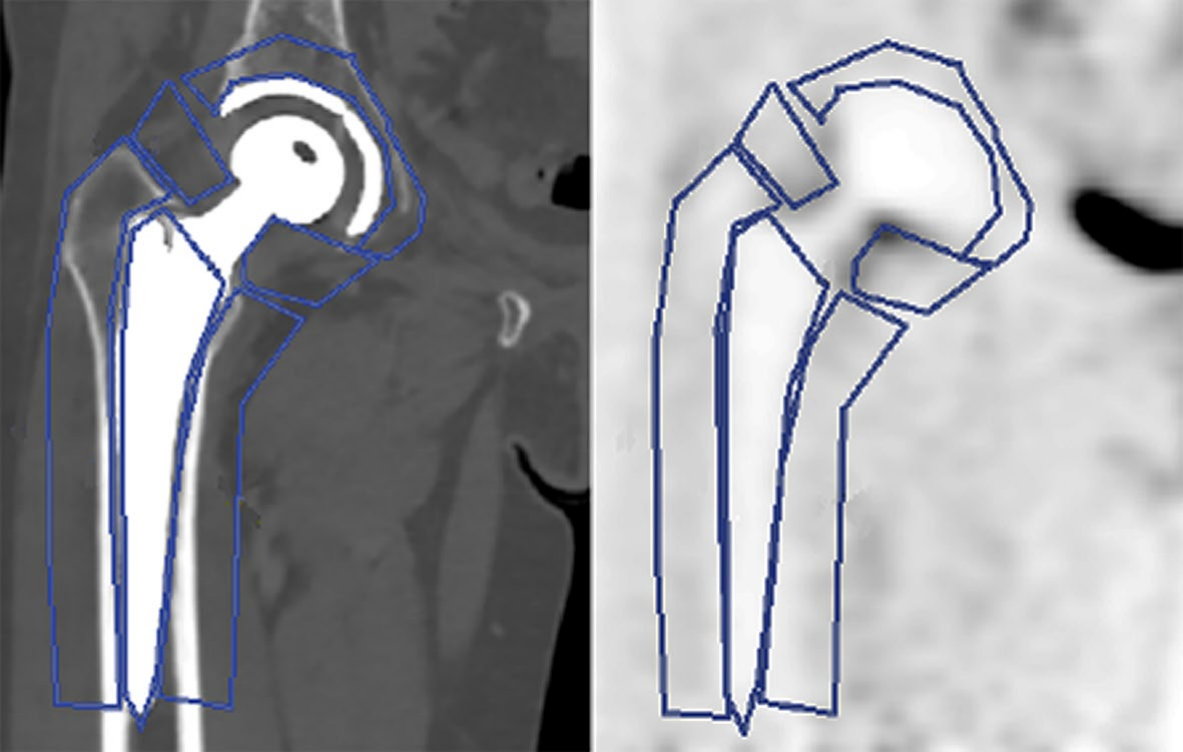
Typical ROIs outlined on corresponding CT and PET images. ROIs correspond to acetabular, medial and lateral neck, medial and lateral diaphysis, and shaft areas. They were drawn in coronal planes through the midplane of the prosthesis
